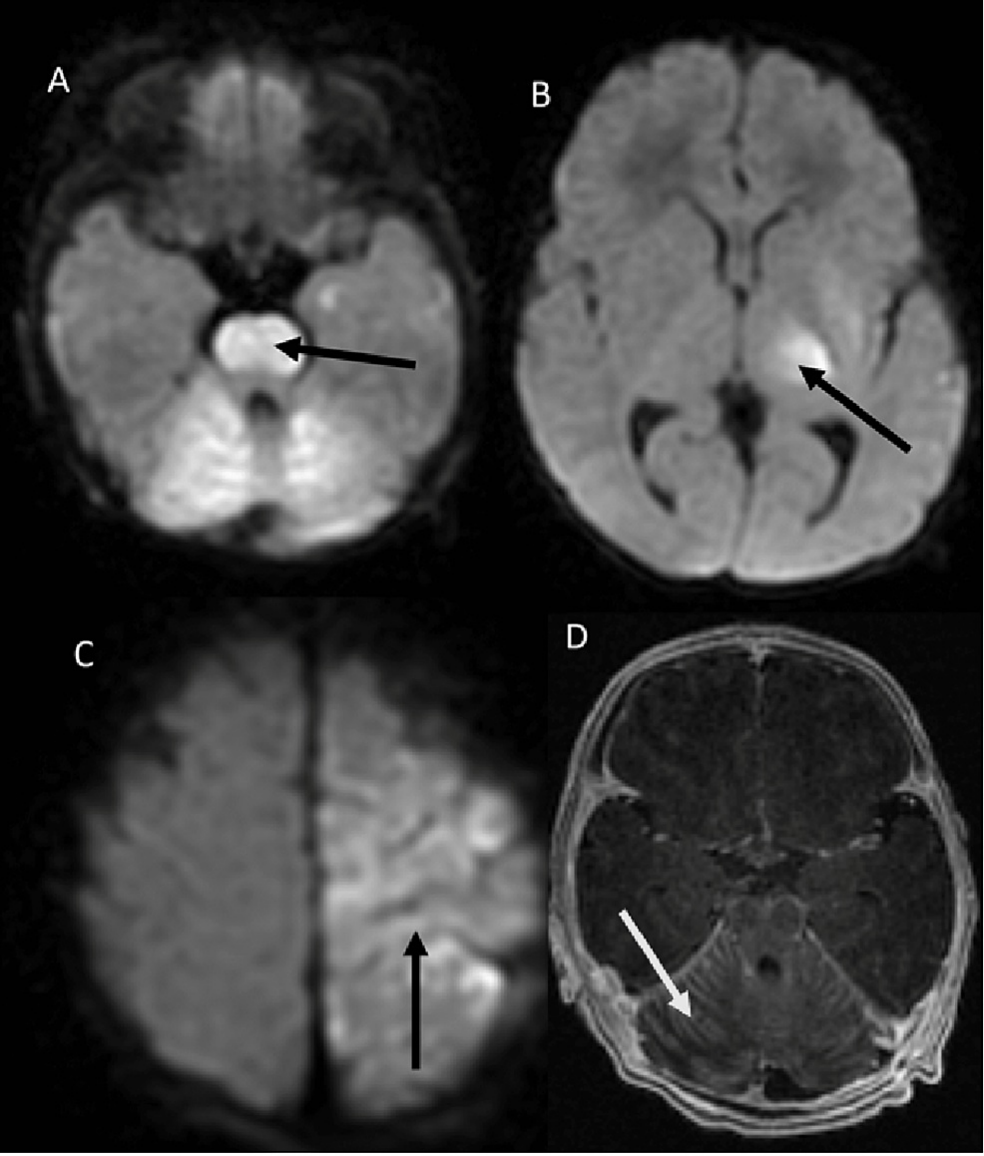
Diffusion-weighted axial MRI images of the brain (A, B, and C) show multiple areas of acute infarcts including the brainstem (arrow in A), left thalamus (arrow in B), and left motor cortex (arrow in C). Contrast-enhanced T1-weighted axial MRI image of the brain (D) at the level of the cerebellum shows extensive leptomeningeal enhancement (arrow in D).
